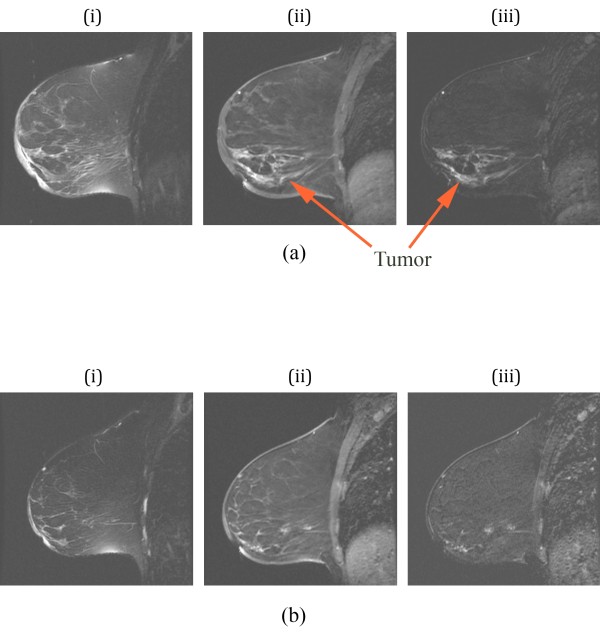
Sagittal MR images of the right breast of a patient with a complete pathologic response: (a) images prior to therapy and (b) at day 85. For each set, (i) is a T2-weighted image, (ii) is a contrast-enhanced image from a SPGR sequence, and (iii) is a subtraction image between (ii) and a pre-contrast baseline, respectively. MR, magnetic resonance; SPGR, spoiled gradient recalled.
